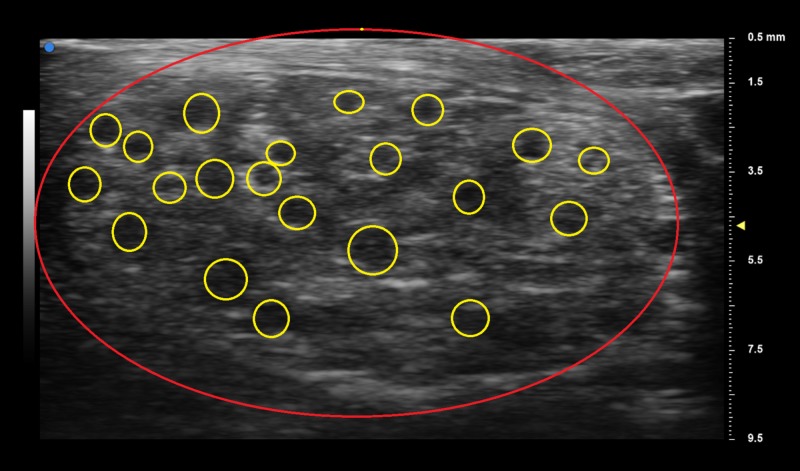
Ultra-high-frequency ultrasound (UHFUS) showing the lipofibromatous hamartoma of the median nerve The red line indicates the circumference of the median nerve; yellow circles surround the nerve fascicles
